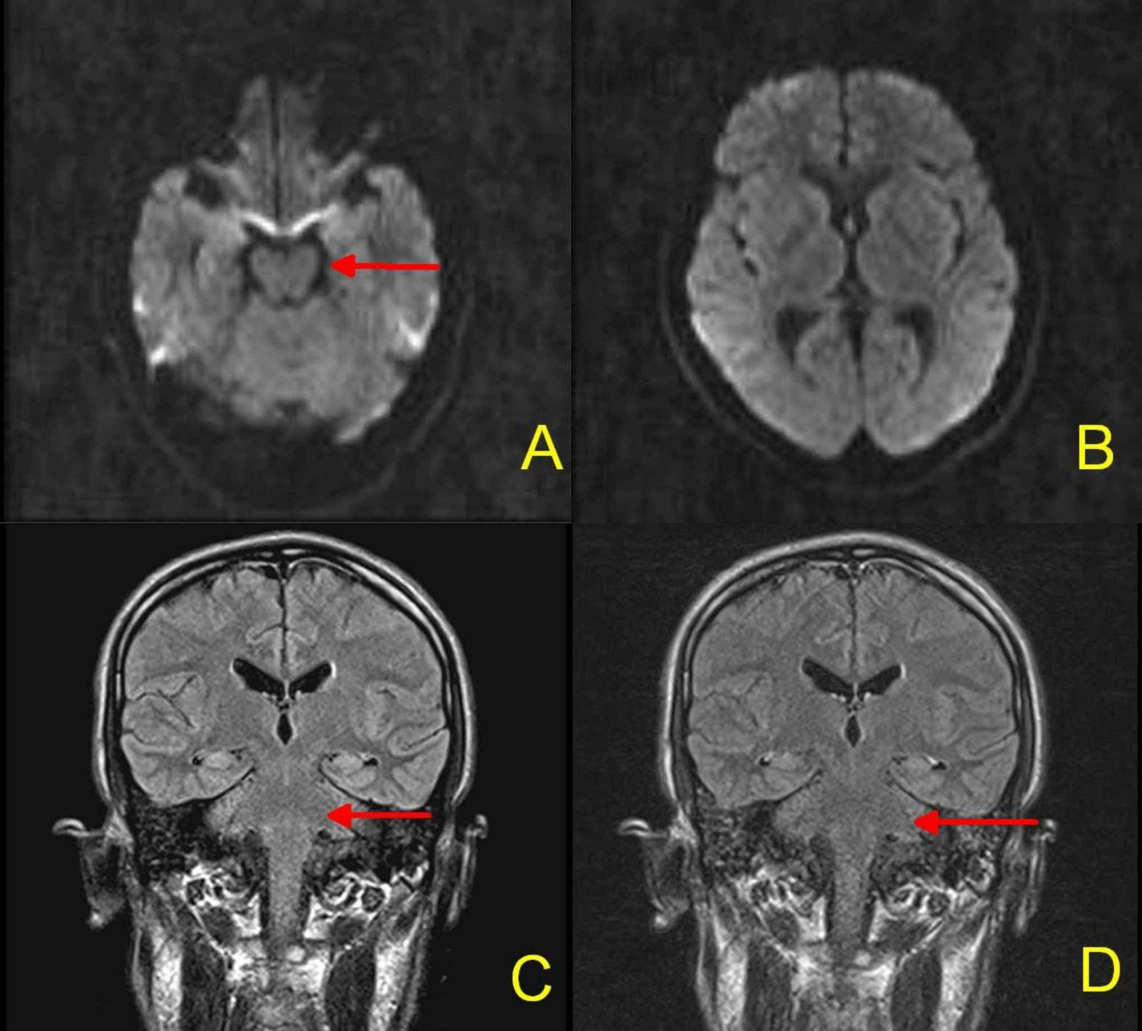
MRI Brain: No acute ischemic stroke on DWI sequences (Figure 1A, 1B). No abnormal signals on pre-contrast ( Figure 1C) or post-contrast FLAIR sequence (Figure 1D). Red arrows indicate brainstem, which is normal MRI = Magnetic resonance imaging; DWI = Diffusion-weighted imaging; FLAIR = Fluid-attenuated inversion recovery
